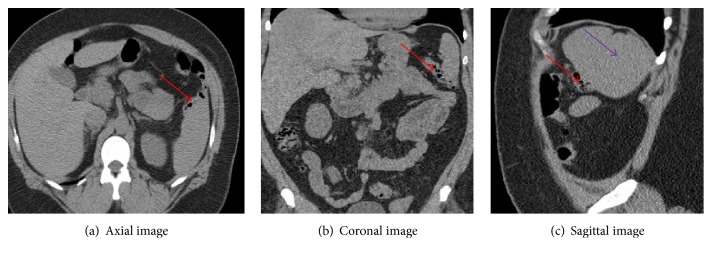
CT, 06/06/12: diverticula abutting the splenic capsule of the lower pole (red arrows) with splenomegaly (purple arrow).
